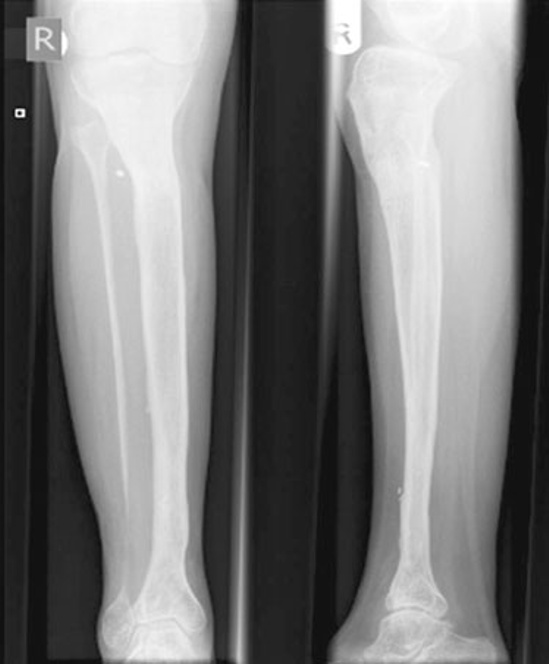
Plain radiographs at 1-year follow-up showing a fully healed corticotomy site with filling-in of the previous scalloping
